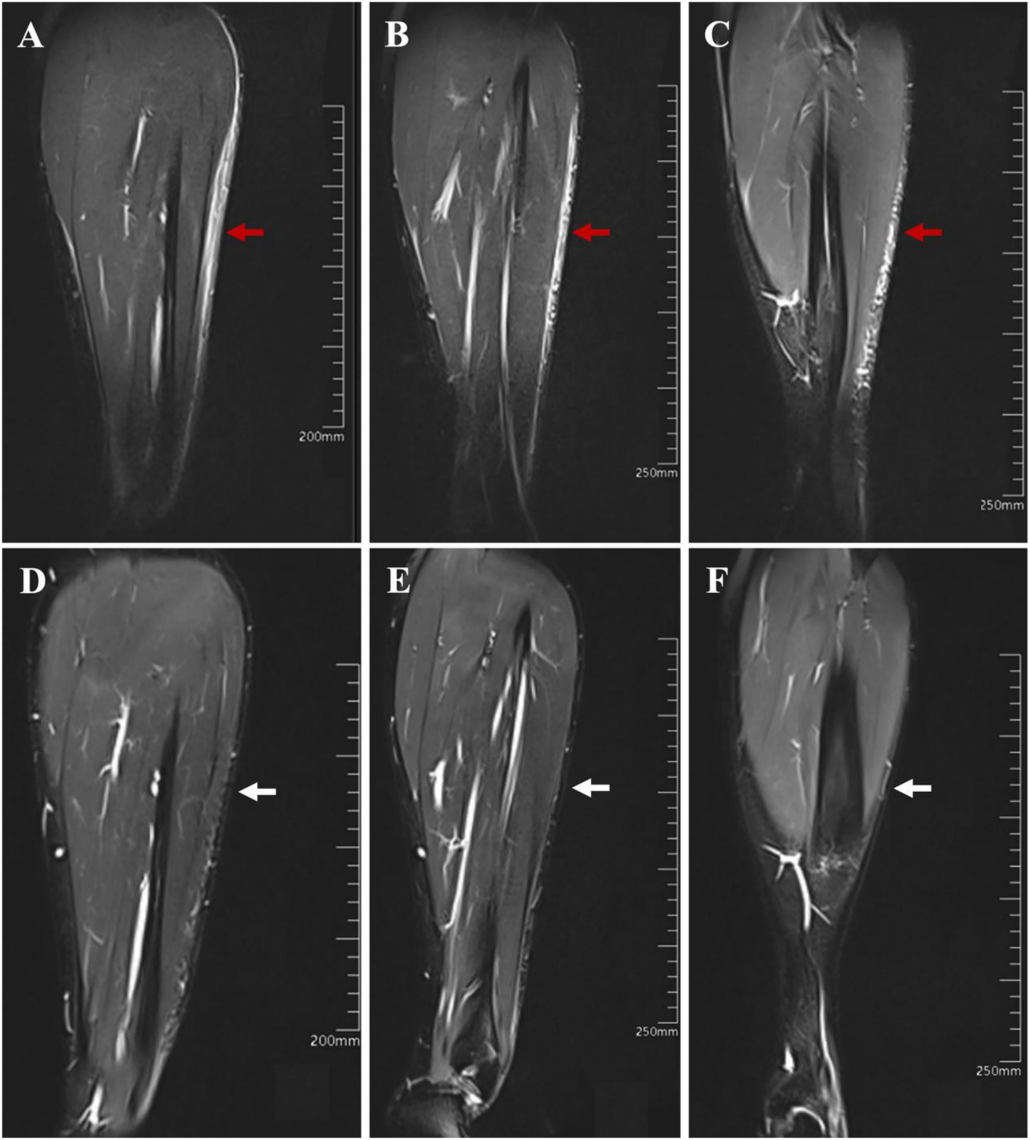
Magnetic resonance imaging findings of the left lower leg. (A–C). Baseline MRI scan (Baseline, Month 0) showing abnormal signals (high signal on fat-suppressed images) in the subcutaneous fat layer and blurred muscle spaces, indicating active Deep Morphea (red arrows). (D–F). Follow-up MRI scan (Month 6) showing significant reduction of high signal shadows in the subcutaneous fat layer (marked reduction, white arrows), consistent with decreased inflammatory activity after 6 months of tocilizumab-based therapy.
